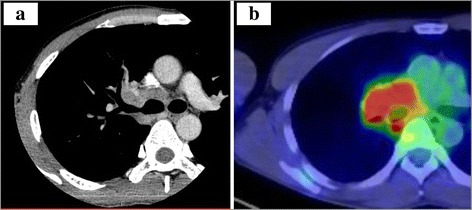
Preoperative imaging studies. Computed tomography of the chest showing the localization of the tumor. The tumor is located in the right hilar. The tumor surrounds the right upper bronchus (a). 18-fluorodeoxyglucose (FDG) positron emission tomography/computed tomography shows moderate FDG uptake within the tumor (b). The *pink-colored* area indicates that the maximum standardized uptake value is 8.1 or more
